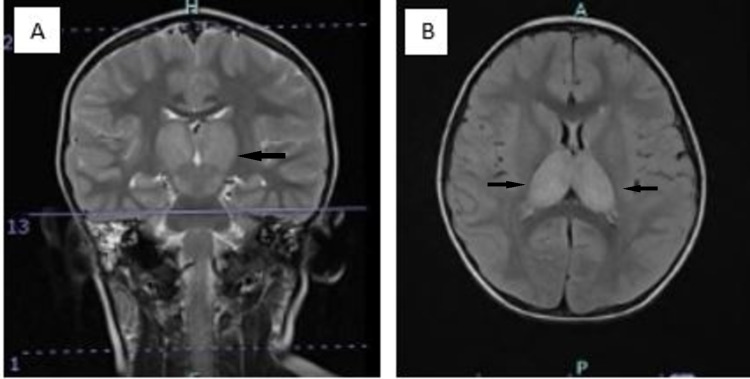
Coronal T2 (A) shows abnormal signal intensities in bilateral thalami. No other abnormal signal intensities. Axial FLAIR (B) sequence shows a non-suppressed hyperintense signal in both thalami.
